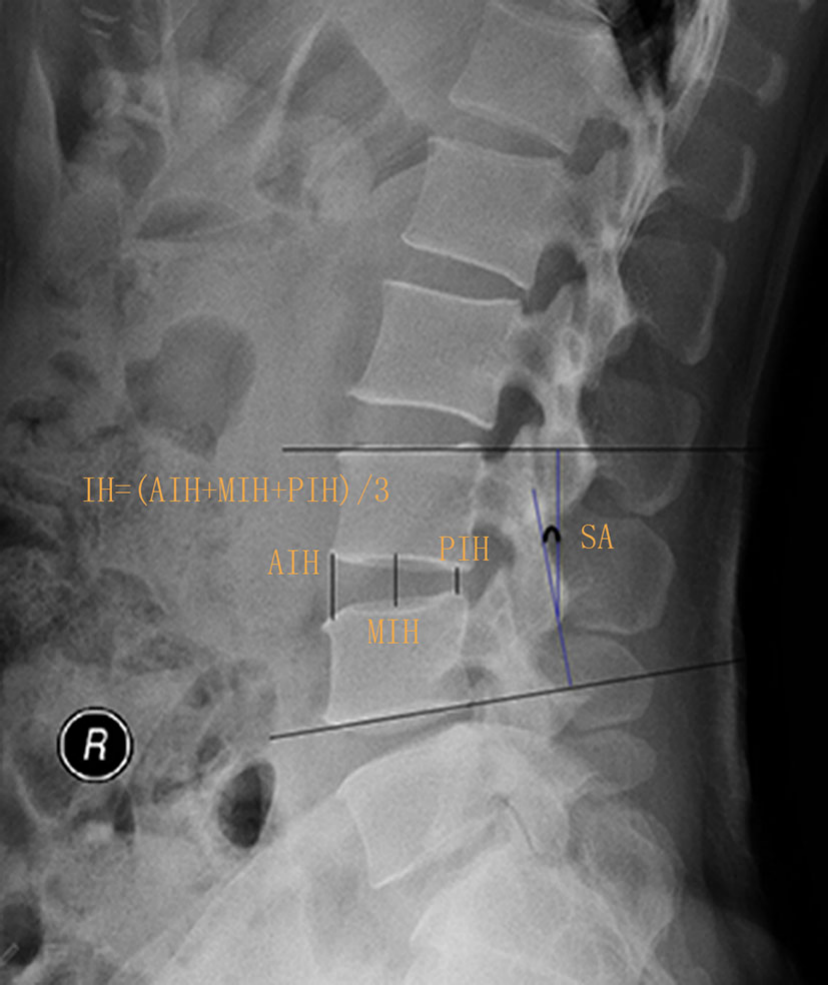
Methods to measure intervertebral space height and segmental angle. IH = (AIH + MIH + PIH)/3. *AIH* anterior intervertebral space height; *MIH* middle intervertebral space height; *PIH* posterior intervertebral space height. SA, between the superior endplate of upper vertebral and inferior endplate of lower vertebral of fused segment on neutral lateral lumbar plain film
